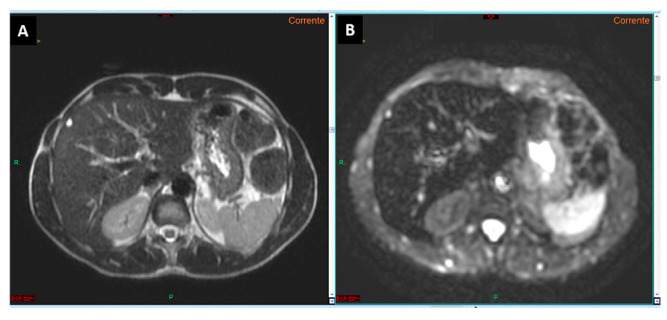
T2-weighted image (A) and diffusion-weighted image with a b-value 0 (B). It is evident that the diffusion-weighted image (B) corresponds to the T2-weighted imaging (A) in terms of signal intensity, as demonstrated by the signal (hyperintensity) of the small cyst located in the liver segment IV.
